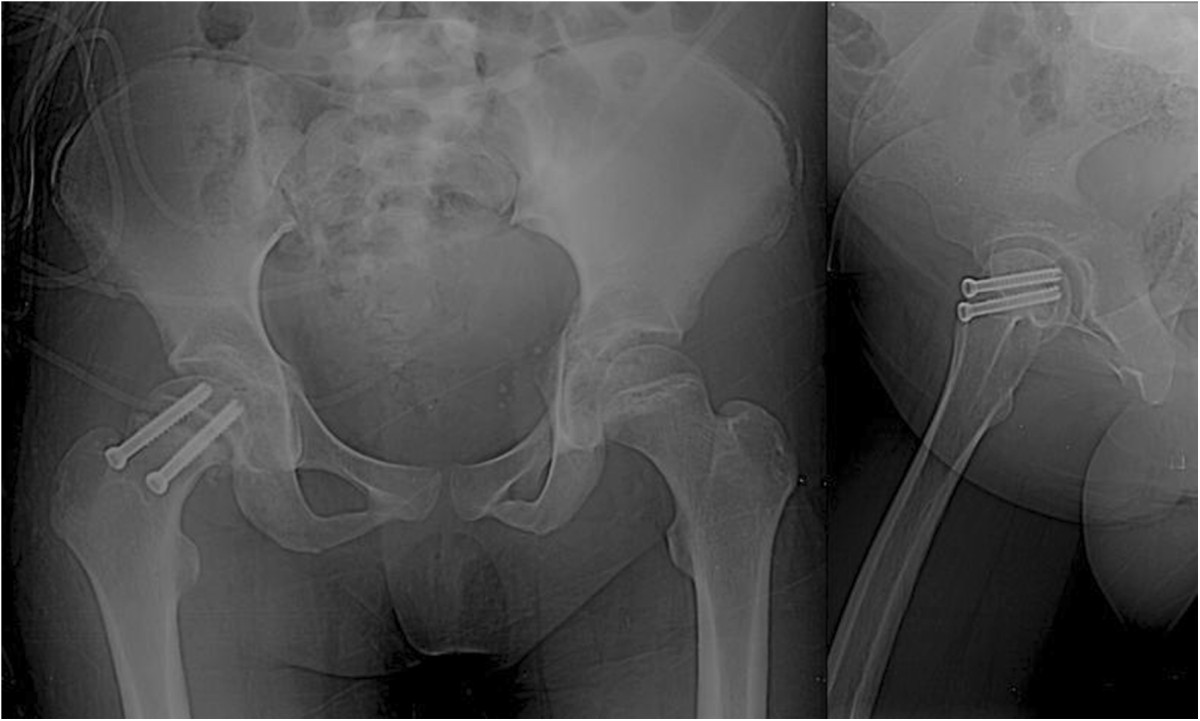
Post-operative radiograph of the patient’s right hip, where reduction by two cannulated 4.0 screws at a 6° Southwick angle can be seen.
